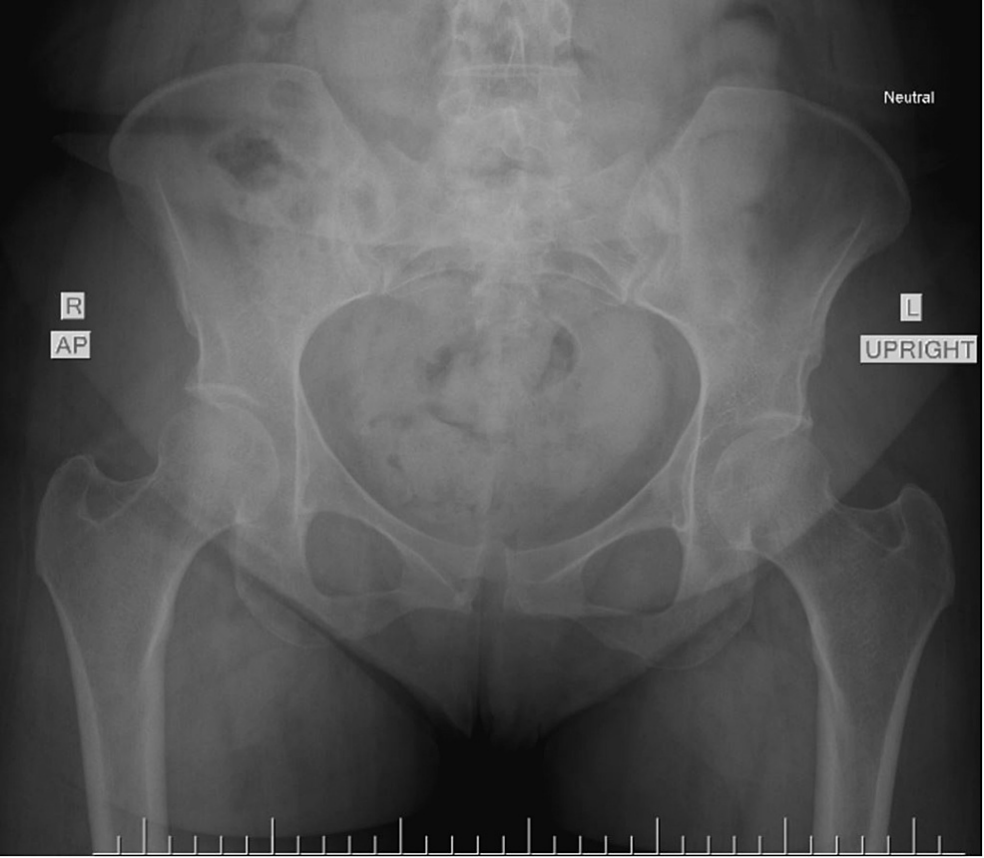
Neutral anteroposterior (AP) radiographic view of the pelvis in our patient
